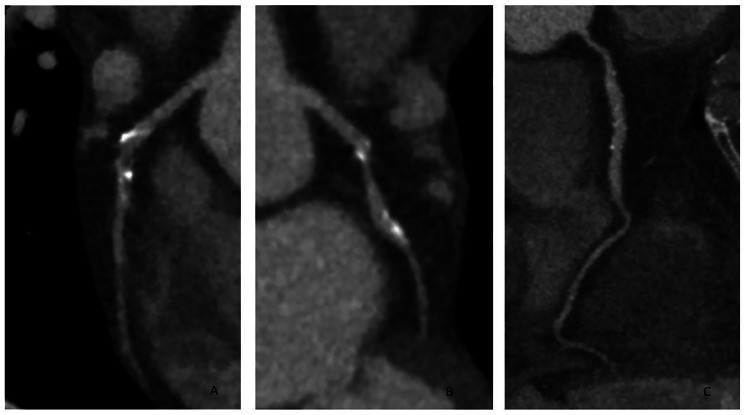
Figure 3. Legend: CT coronary angiography. No significant luminal stenosis was identified. (A) LAD, (B) LCx,C. RCA
